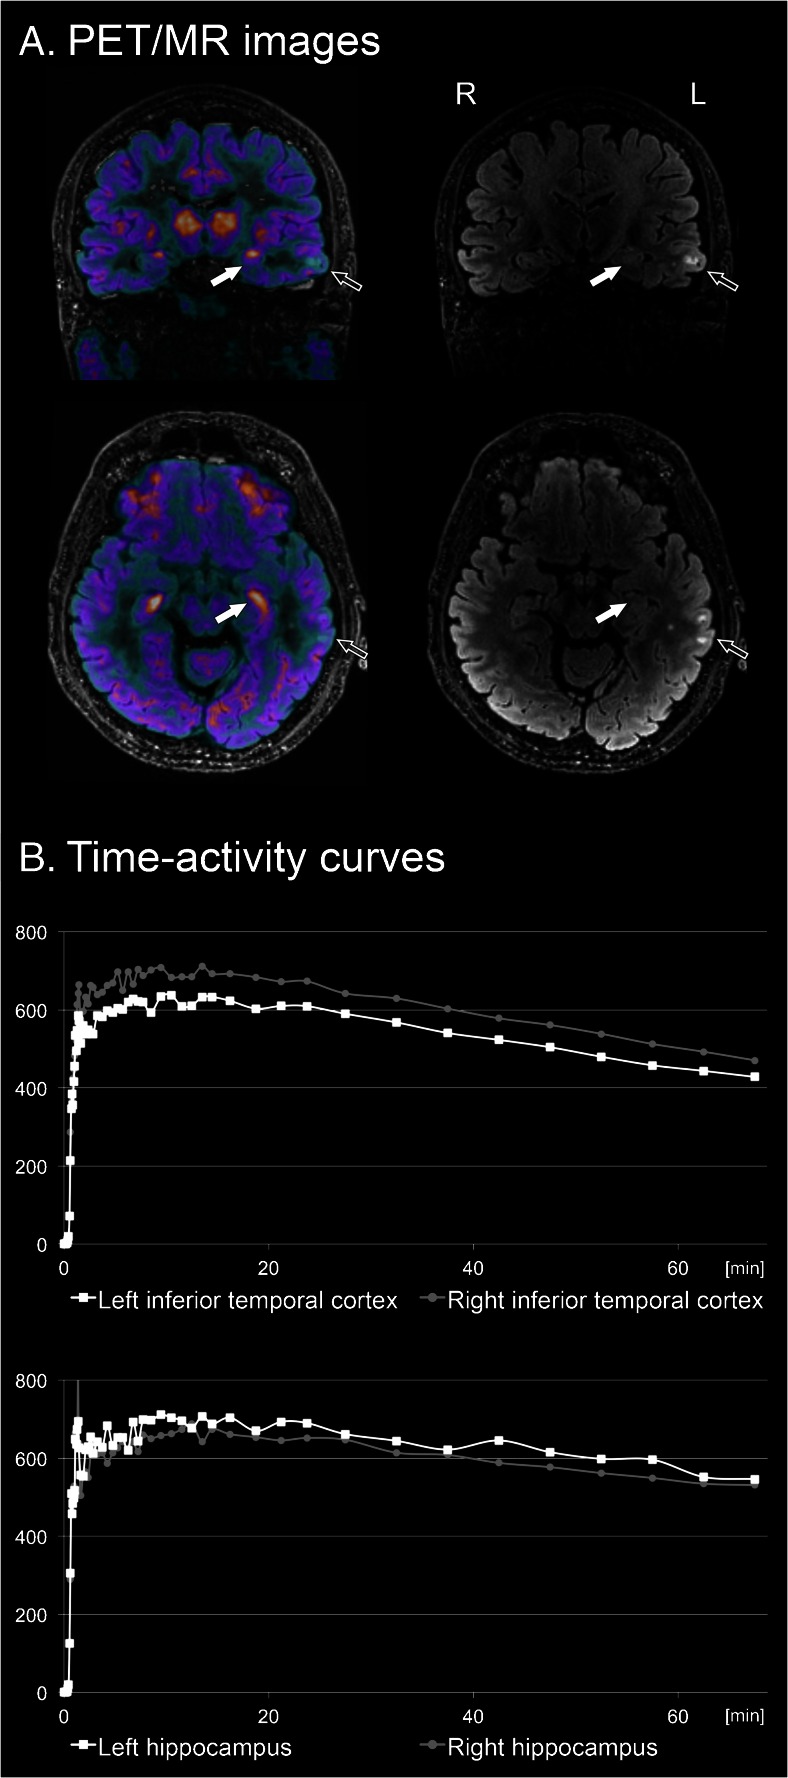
[^18^F]GE-179 PET/MR scan in a 57-year old temporal lobe epilepsy patient with signal abnormality in left inferior temporal cortex. a PET/MR fusion image (*left*) and MR FLAIR sequence (*right*) displaying parts of the lesion (*empty arrow*) and the hippocampus (*full arrow*). b Bilateral comparison of time-activity curves (*TACs*) in the inferior temporal cortex (*above*) and the hippocampus (*below*). Both visual analysis and TACs show reduced tracer uptake in the lesioned temporal cortex and slightly increased uptake in the ipsilateral hippocampus. Hypothetically, the extralesional increase of NMDA-receptor activation in the ipsilateral hippocampus could point to ongoing epileptogenesis and prospective studies will be needed to prove this assumption
